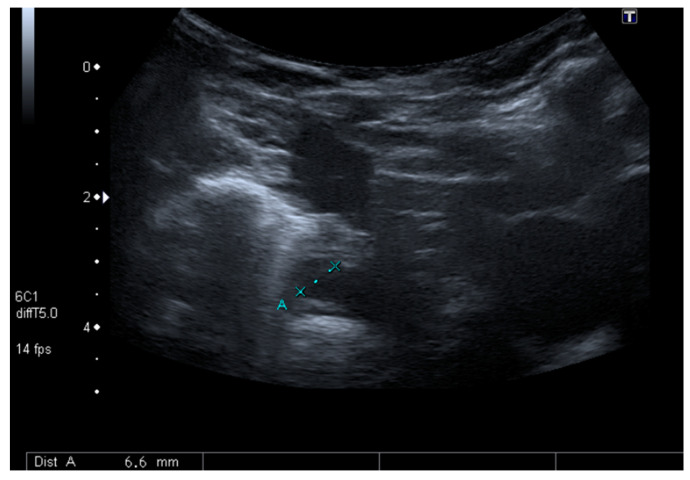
Follow up mediastinal ultrasound.
